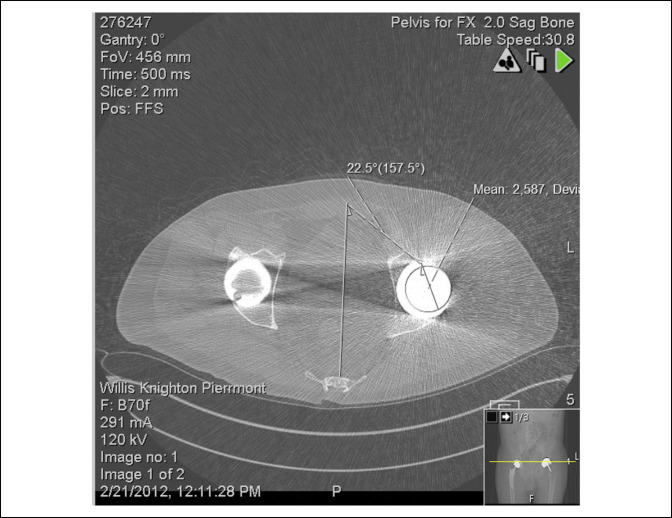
CT scan revealing the left Birmingham hip resurfacing cup anteversion angle of 22.5°.
